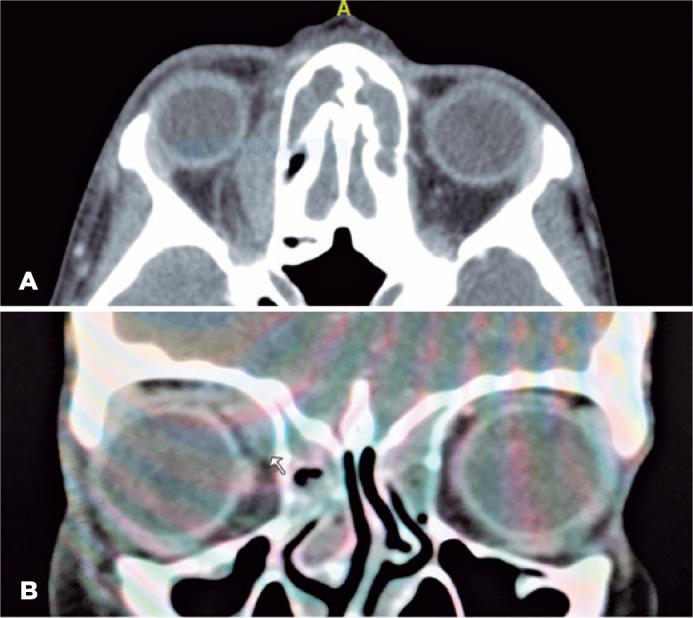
CT scan of the orbits upon admission. A) Axial superior orbital
